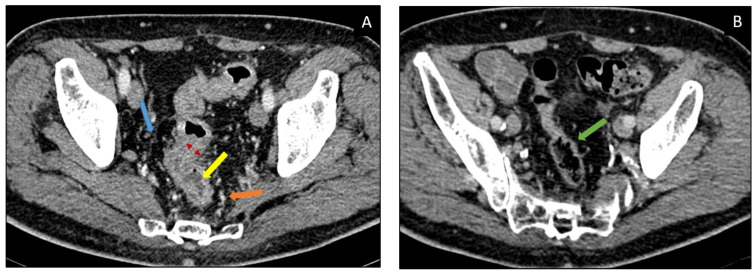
73-year-old patient with lung squamous cell carcinoma under Pembrolizumab. (A) Axial post-contrast CT image demonstrating colitis after 8 weeks of ICI administration, with diffuse bowel wall thickening (red arrowheads) and mucosal hyperemia (yellow arrow), at the level of sigma and rectus; also associated with peri-visceral fat stranding (blue arrow) and local vascular engorgement (orange arrow). (B) Axial post-contrast CT image showing colitis resolution (green arrow) after Pembrolizumab discontinuation and corticosteroid administration.
